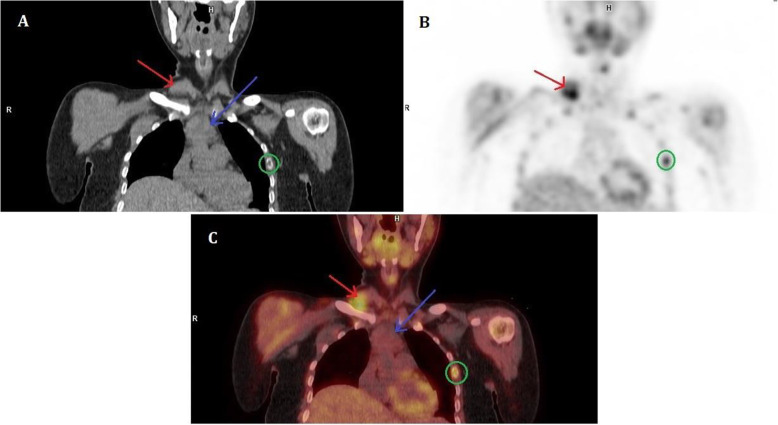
^18^F-FDG PET/CT: A coronal computed tomography image, B coronal PET images, and C fused PET/CT coronal image. There are FDG-avid right supraclavicular lymph node (red arrows), left fourth rib lytic lesion (green circles), and non-FDG-avid large anterior mediastinal soft tissue mass (blue arrows)
